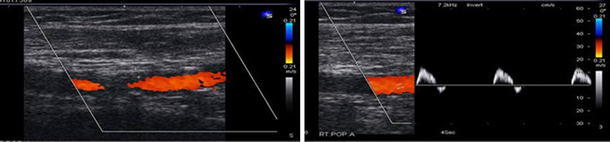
Doppler sonography. Mild diffuse atherosclerotic plaque changes without significant stenosis, but with decreased peak flow velocity on spectral mode
